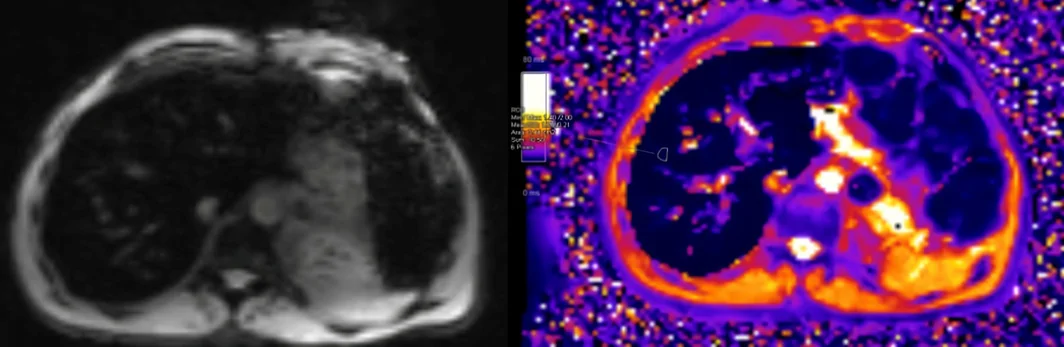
MRI of the abdomen showed iron deposition in the liver. Liver T2* showed liver iron loading was 1.5 ms, corresponding to 8–15 mg/g, which indicates severe liver iron overload [4].
